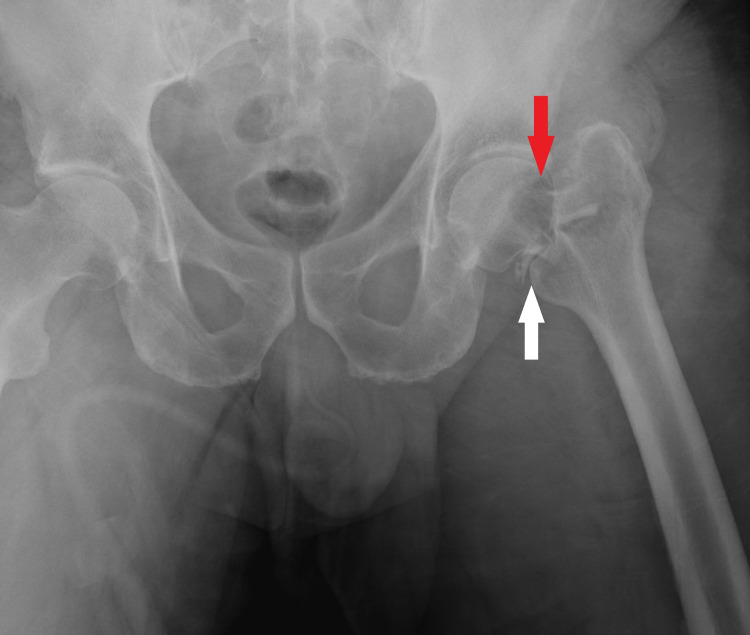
X-ray revealing comminuted left femoral neck fracture (White Arrow) with a possible suspicious lytic lesion (Red Arrow) on the femoral neck
